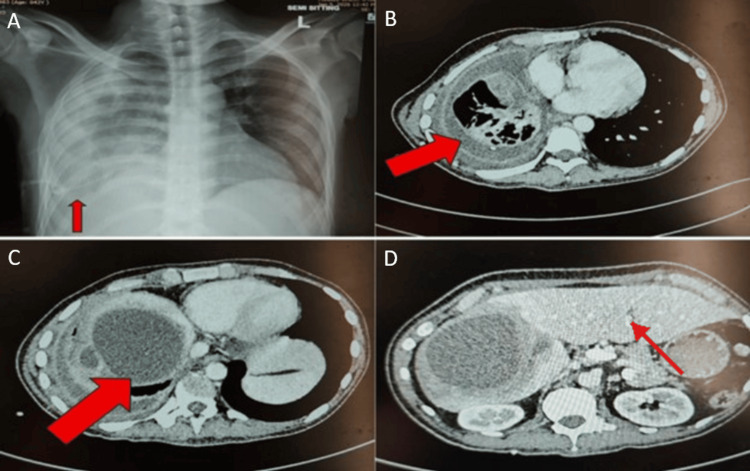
Radiological findings demonstrating pleural empyema secondary to complicated hepatic cysts (A) Semi-sitting anteroposterior chest radiograph showing right basal haziness with a right-sided intercostal chest drain in situ (arrow). (B) Contrast-enhanced axial CT of the chest demonstrating a right-sided heterogeneous pleural collection with internal air-fluid levels (arrow) and parenchymal involvement, consistent with empyema thoracis. (C) Contrast-enhanced axial CT at the level of the upper abdomen revealing a large complex multiloculated hepatic cyst with internal septations and a dependent fluid level in the right lobe of the liver (arrow). (D) Contrast-enhanced axial CT at the level of the mid-abdomen showing a right hepatic hypodense lesion without active enhancement, in keeping with a complicated simple hepatic cyst.
